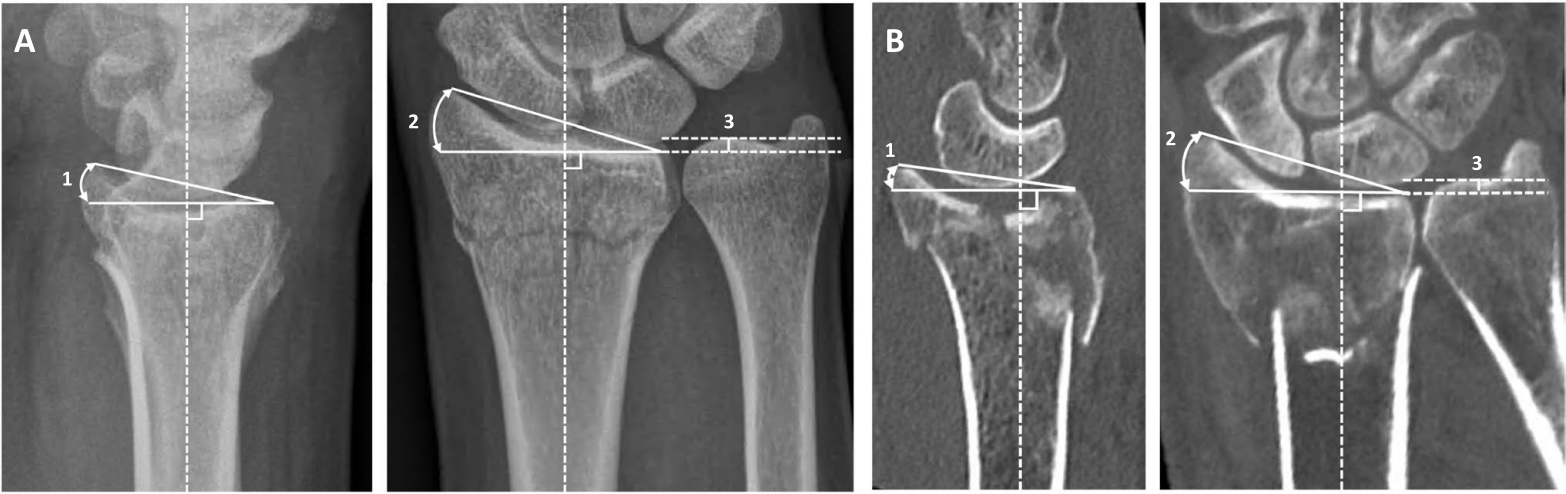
Examples of measurements on radiographs and CT scans: A (1) Angulation, (2) inclination, and (3) positive ulnar variance on radiographs; B (1) Angulation, (2) inclination, and (3) positive ulnar variance on CT scan
